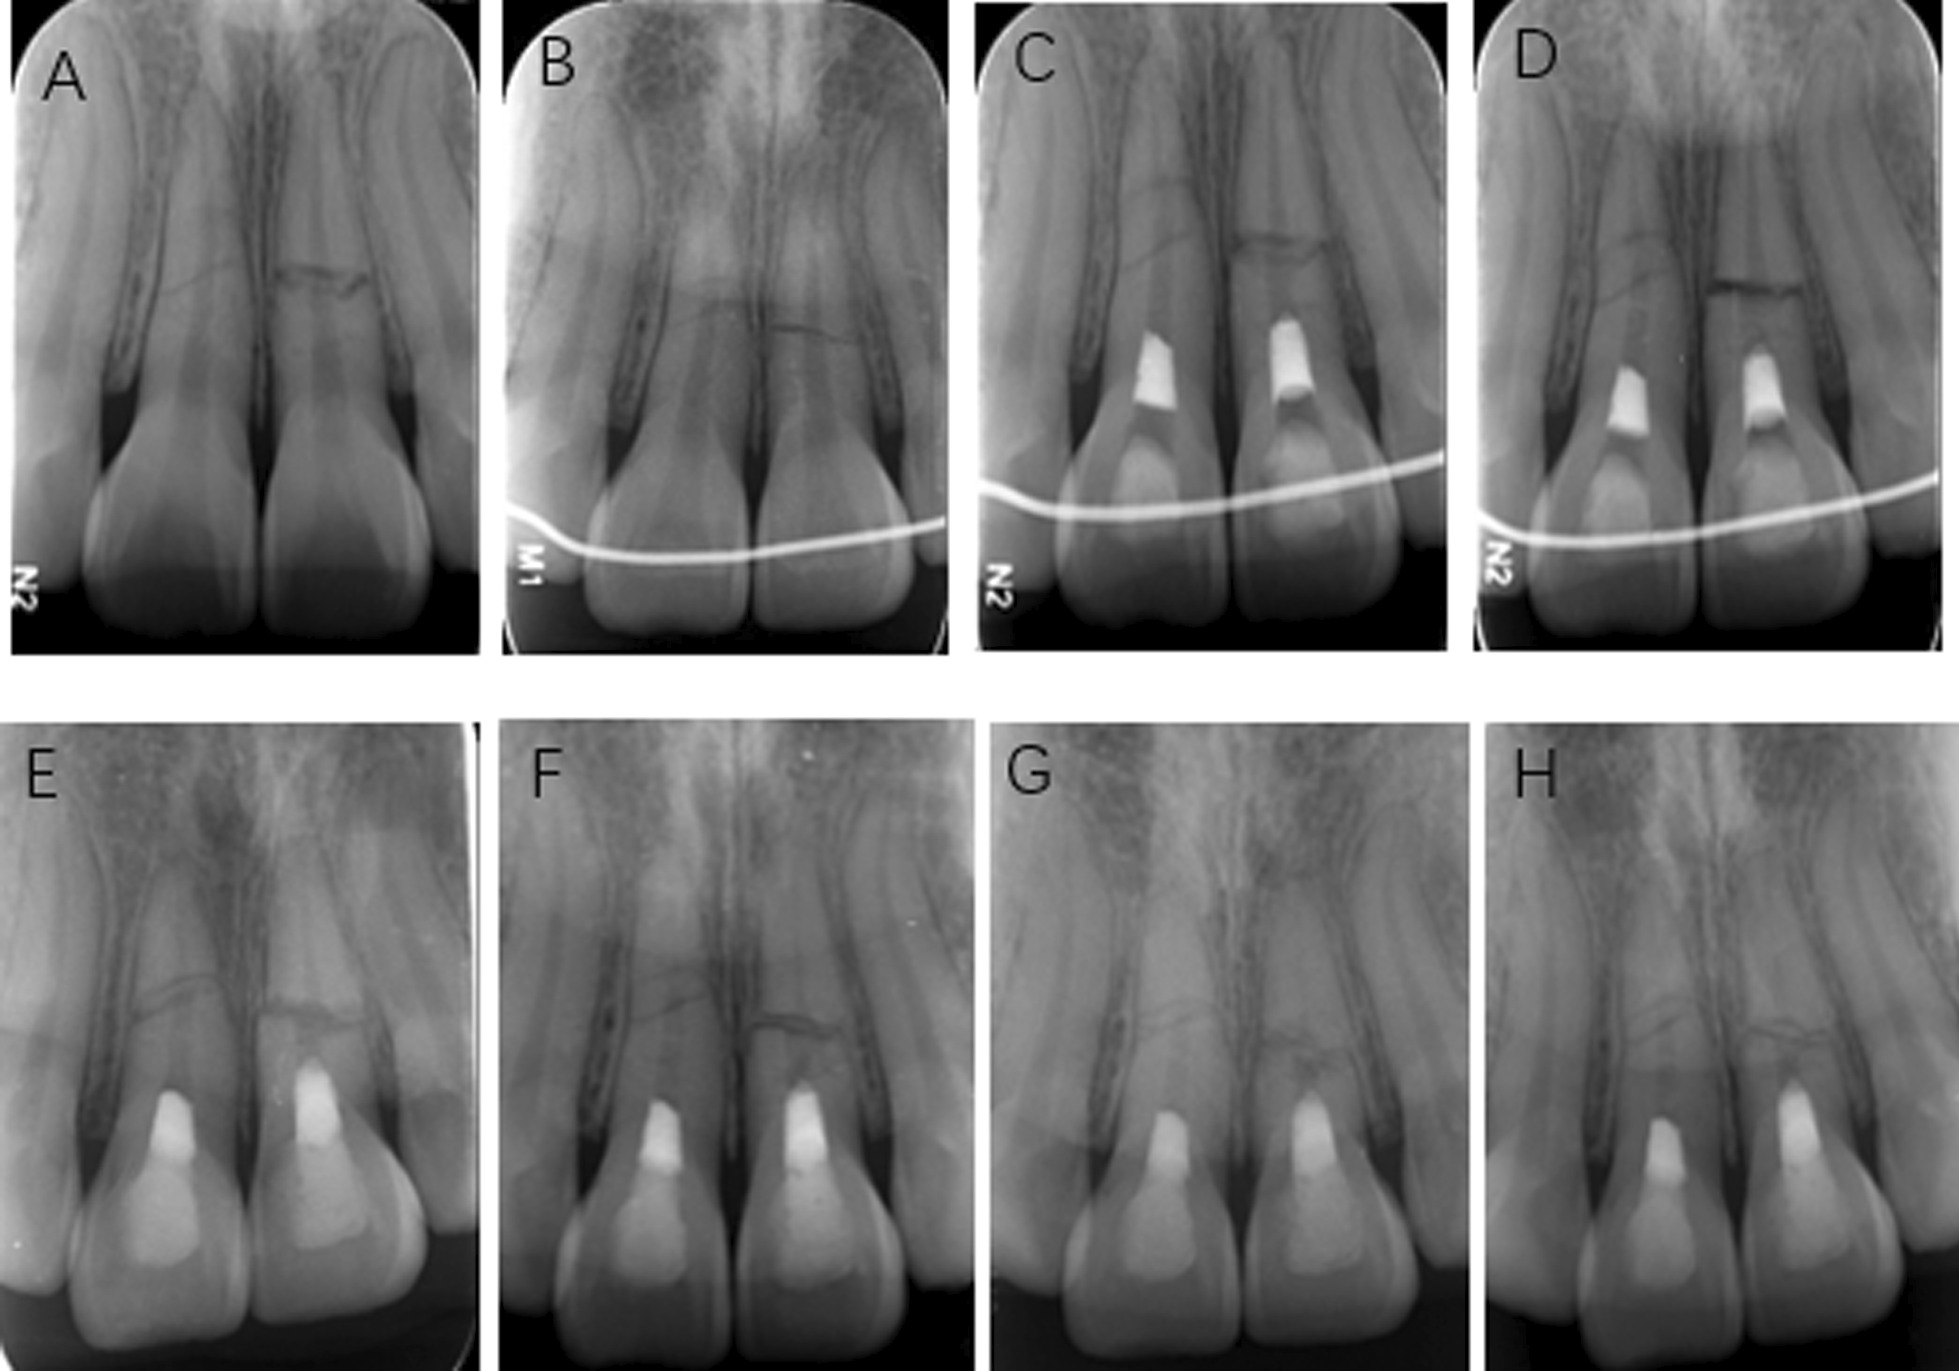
A Periapical radiograph of teeth #11 and #21 after trauma. The roots were completely formed. Multiple horizontal fractures in the middle third of the roots of teeth #8 and tooth #9 were evident. B Preoperative periapical radiograph before REPs. C Postoperative periapical radiograph after completion of REPs. D Postoperative periapical radiograph at the 1-month follow-up. No radiolucent lesion developed at the fracture line. E Postoperative periapical radiograph at the 6-month follow-up. Progressive healing of the horizontal root fracture by formation of hard tissue between fragments of tooth #21. F Postoperative periapical radiograph at the 14-month follow-up. More calcified in the fracture lines and root canal space of apical fragment of both teeth. G Postoperative periapical radiograph at the 25-month follow-up. Further healing of horizontal root fracture by formation of hard tissue, and calcification in the apical fragments of both teeth. H Postoperative periapical radiograph at the 36-month follow-up. The formation of calcified tissue continued between fragments of tooth #21 and coronal part of tooth #11. Partial canal obliteration is also evident in the coronal fragment. There is near complete calcification of the pulp space in the apical fragment. There was no evidence of a periapical lesion for either tooth
